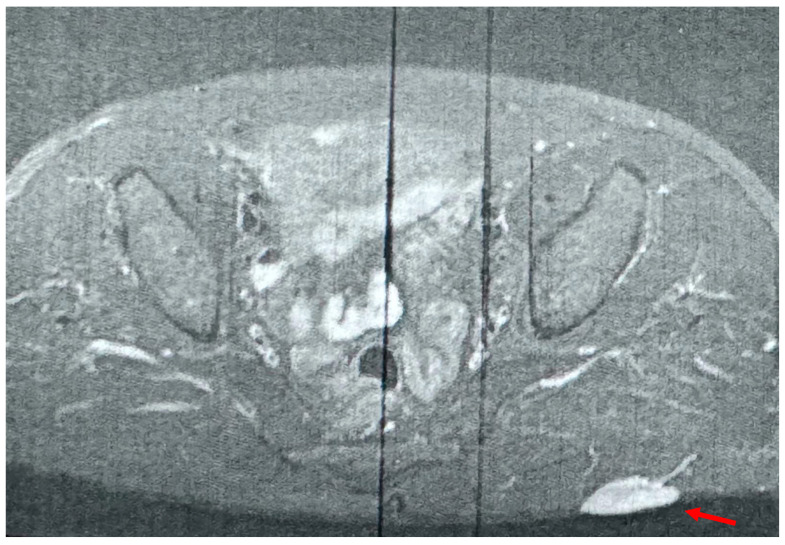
Due to the lesion being highly suspicious of malignancy, an MRI was proposed to identify its local growth and potential characteristics. The radiology report from the MRI characterized the lesion (red arrow) as benign, suggesting that it could be a hemangioma.
